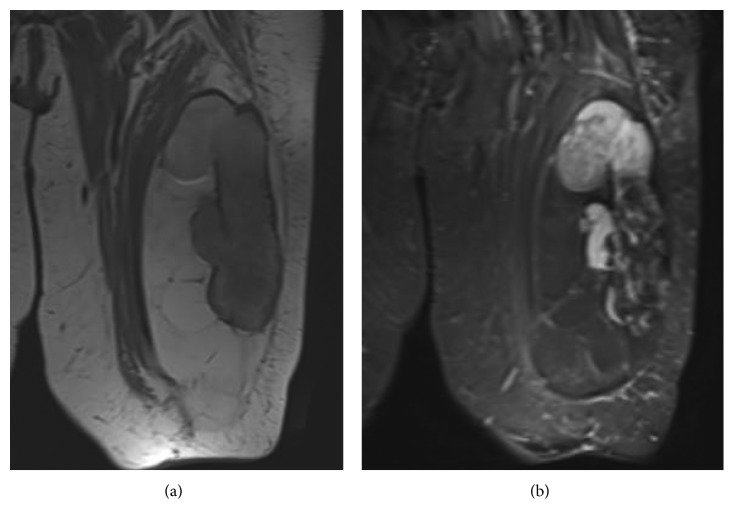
Coronal T1 (a) and STIR (b) MRI of a 30 cm diameter ALT/WDL confirmed by MDM2 FISH which is deep to fascia, contains foci of T2 enhancement, heterogeneity, nodularity, and internal cystic changes.
